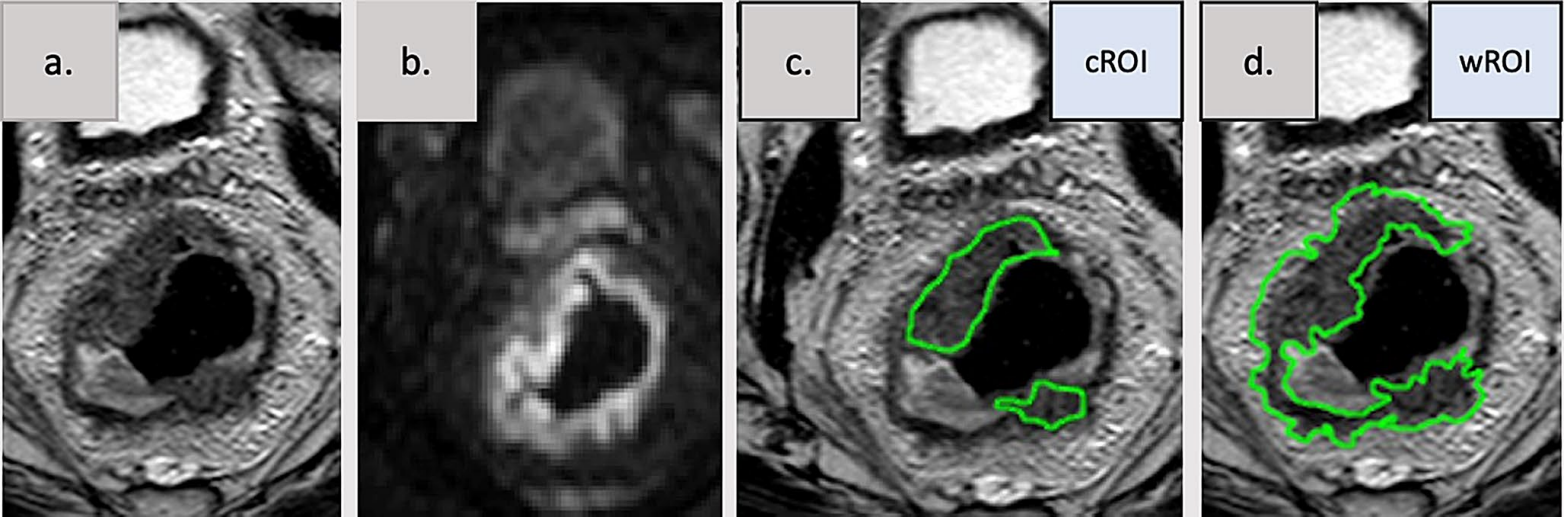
Example of rectal tumor segmentations. Segmentations on axial T2-weighted images (T2w) with a freehand region of interest (ROI). The central (cROI) segmentation includes the tumor bulk excluding the outermost margin and the small extension in the mesorectal compartment, whereas the whole (wROI) segmentation includes tumor bulk and the periphery of the tumor as well as its extensions in the mesorectal compartment. a. T2-weighted images, b. diffusion weighted image (DWI) at the same anatomical position as the T2w, c. T2-weighted images with ROI of central part of tumor (cROI) with segmentation considering the diffusion information, d. T2-weighted images (wROI) including the tumor periphery
